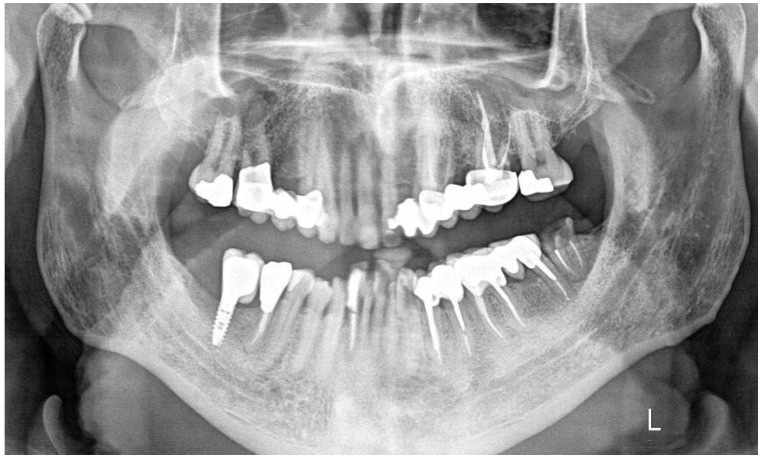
OPT at 5-year recall for teeth #36-#37, implant on #47 and 12 years after crown cementation on tooth #46. Absence of periapical pathology, stable crestal bone level, no evidence of caries or fracture consistent, with stable clinical function.
